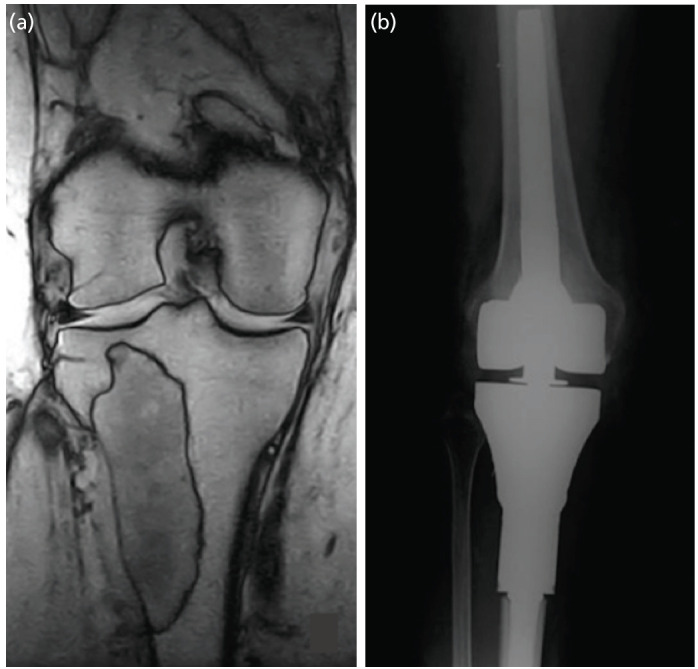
(a) Pre-operative MRI of a fibroblastic osteosarcoma treated with wide massive bone resection and (b) implant of a megaprosthetis.
